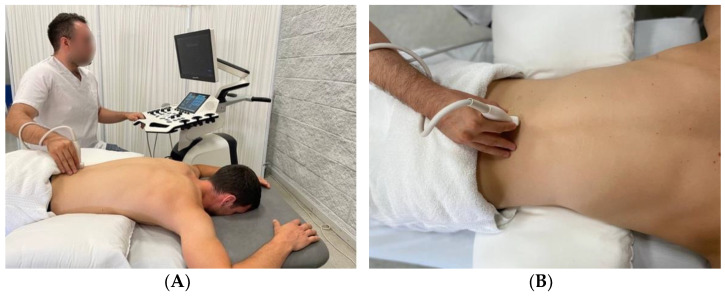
Ultrasound imaging sampling setting. (A) Patient lying prone, sonographer close to the patient and the ultrasound machine; (B) detailed image with the probe parasagittal over the lamina.
